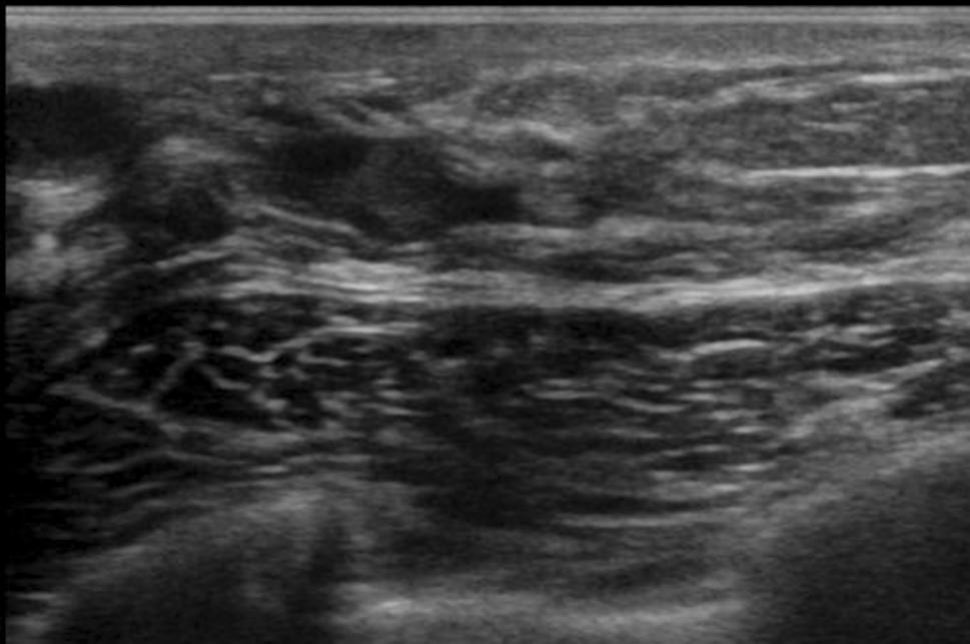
Case of intraductal papilloma. Ultrasound shows that the beginning of the solid component within dilated ducts is precipitous
